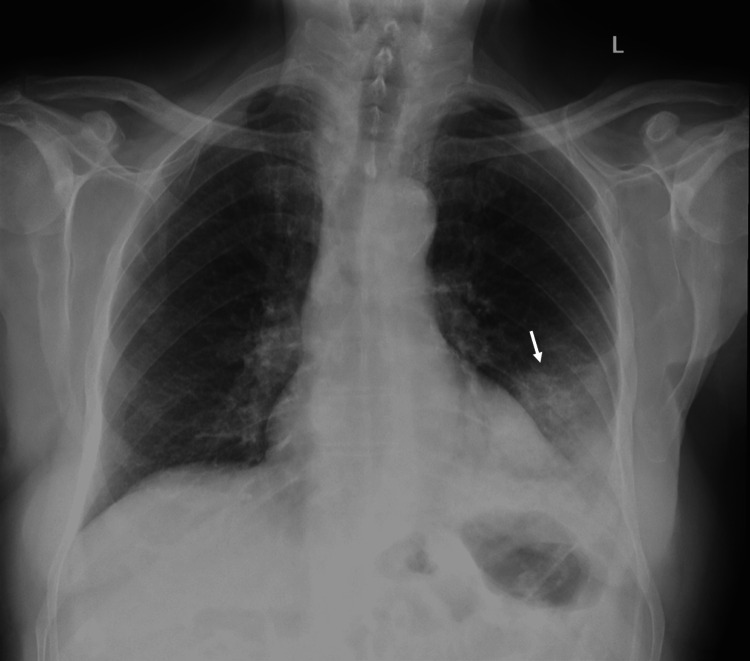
Chest X-ray showing left lower lobe pneumonia.
